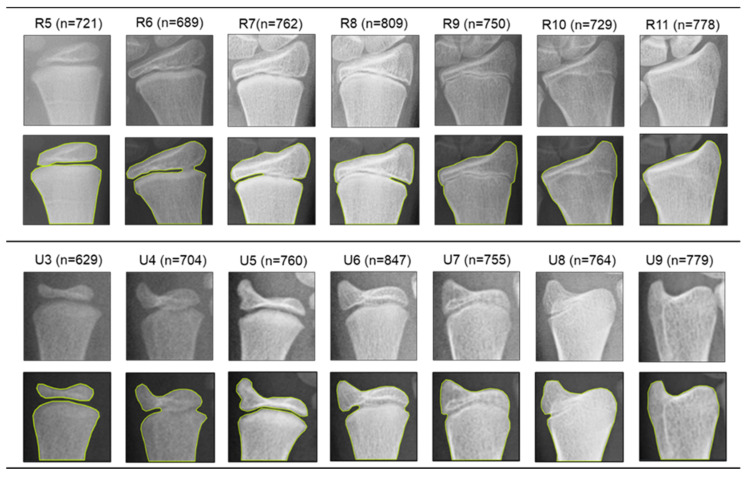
Represented radiographs, segmentation labels, and data size of different ulna and radius maturity stages.
